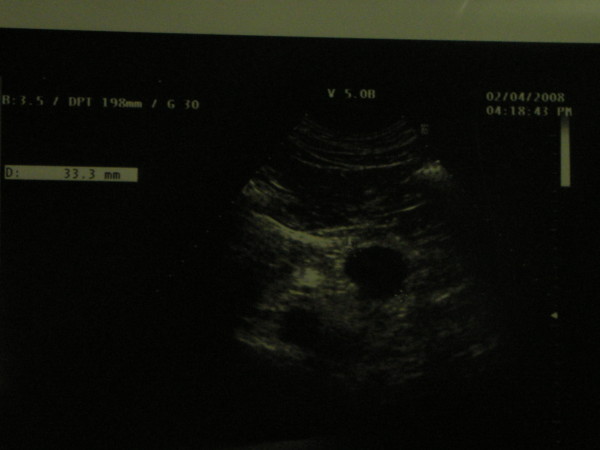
Ultrasonography scan with occasional report of cystic mass of 38 × 28 mm localized in the pancreatic body.
